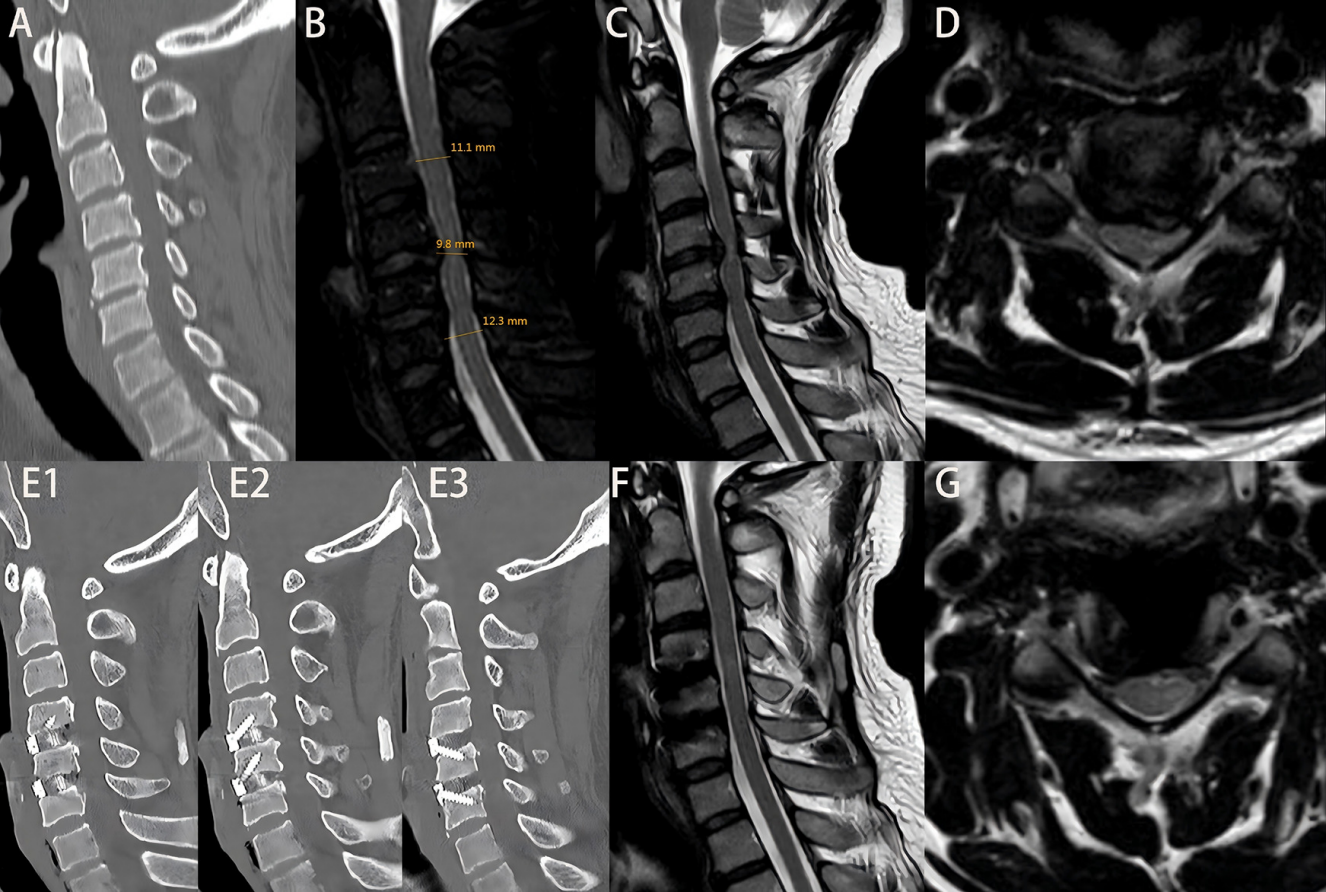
Patient Zhao, admitted 10 days after a traumatic injury, with an initial JOA score of 8. (A) Admission cervical CT scan showing no fracture or dislocation. (B) Measurement of di, da, db, and MSCC values; MSCC calculated to be 16.24%. (C,D) Preoperative cervical MRI showing significant spinal cord compression. On the second day after admission, the patient underwent ACDF at C3–4 and C4–5 levels. The patient was discharged on postoperative day 3. Figures E1–E3: cervical CT at 6 months postoperatively showing proper positioning of screws and interbody fusion device. (F,G) Cervical MRI at 6 months postoperatively showing relief of spinal cord compression. At 2-year follow-up, the JOA score was 14.
